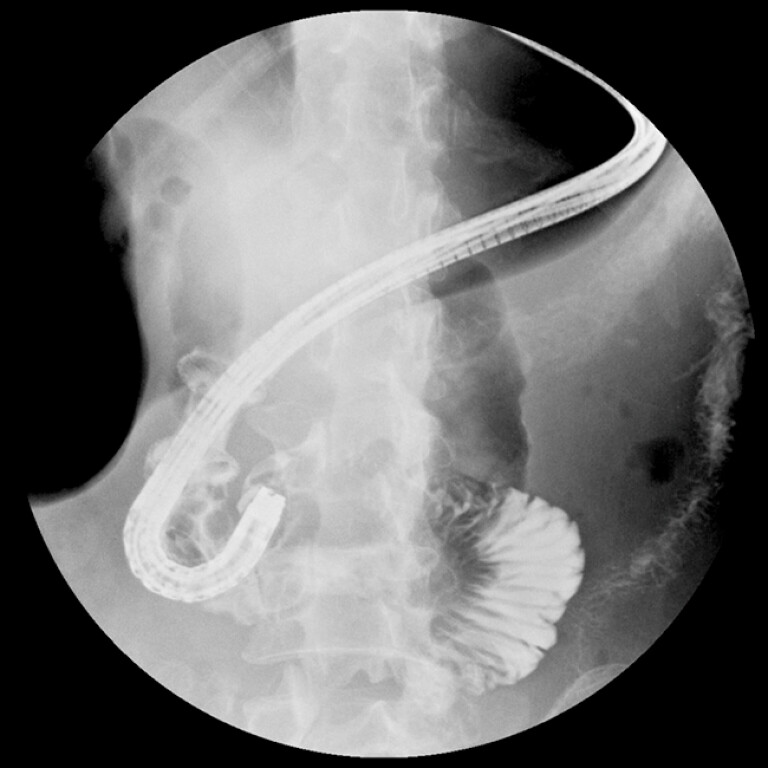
Radiography showed segmental narrowing of the efferent intestinal lumen.
